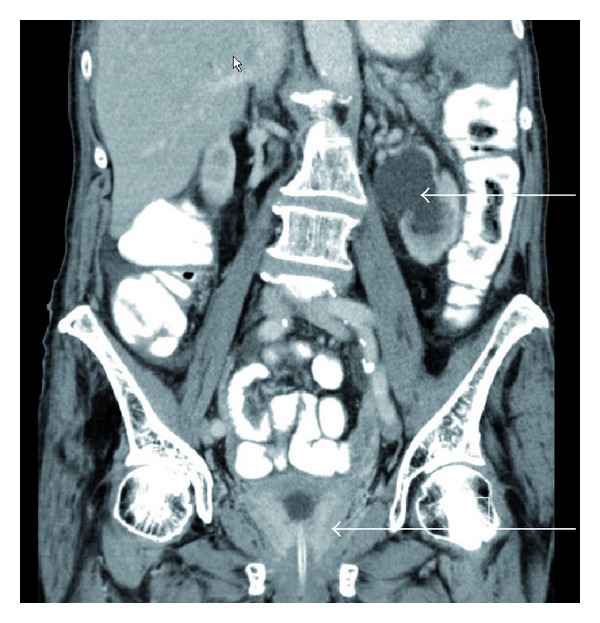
Computed tomography scan of the abdomen and pelvis showing both hydronephrosis (upper arrow) and thickening of the bladder wall (lower arrow), bladder catheter in situ.
